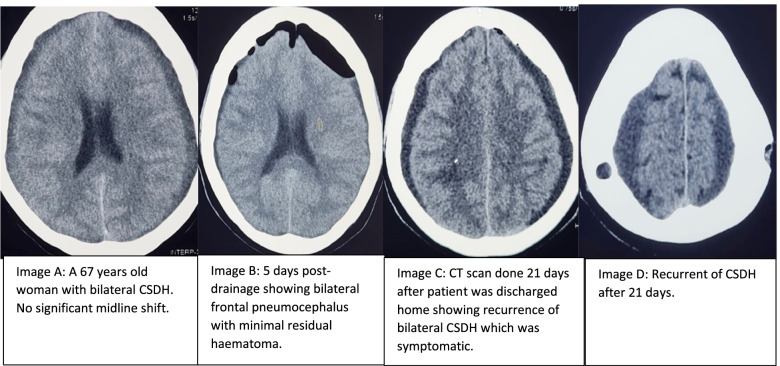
CT scan images of a 67 years old woman with bilateral CSDH before and after burr-hole and drainage surgery
